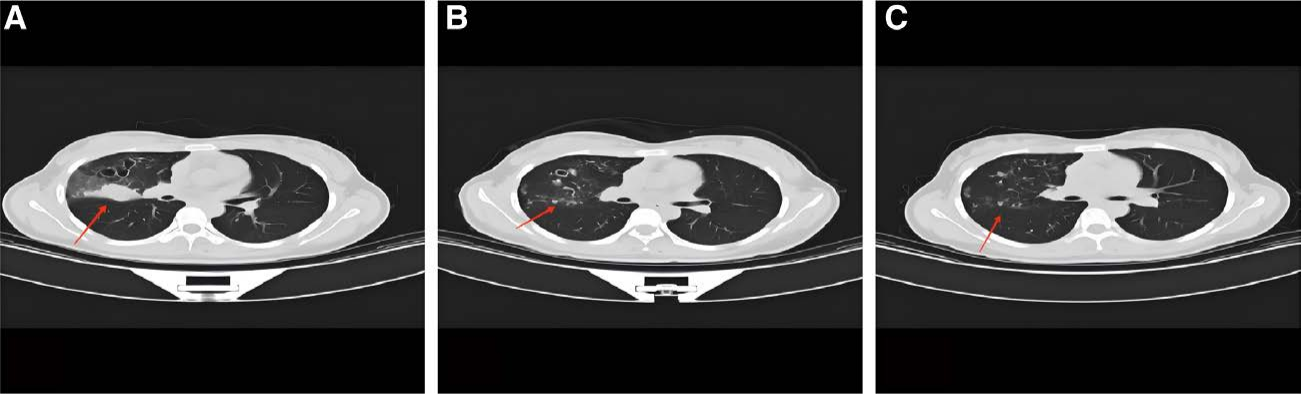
Serial chest computed tomography (CT) manifestations of pulmonary paragonimiasis before and after treatment. (A) Chest CT on September 9, 2022 (admission): patchy, nodular, and striated opacities in the right upper and middle lobes, with heterogeneous density, ill-defined margins, localized pleural adhesion, and multiple cavitary lesions. (B) Chest CT on November 26, 2022 (post-praziquantel treatment): most right lung lesions showed calcification; cystic lucencies were noted in the right middle lobe, with a significant reduction in lesion extent compared to (A). (C) Chest CT on February 13, 2023 (6-month follow-up): complete closure of the cavitary lesion observed in (A). CT = computerized tomography.
